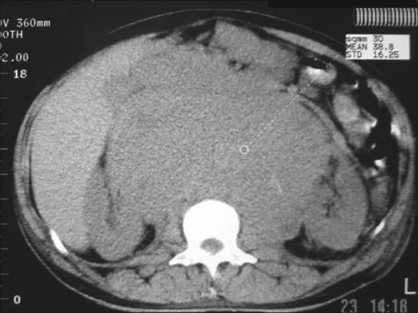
Patient 2: Abdominal computed tomography (CT) revealing large retroperitoneal mass
